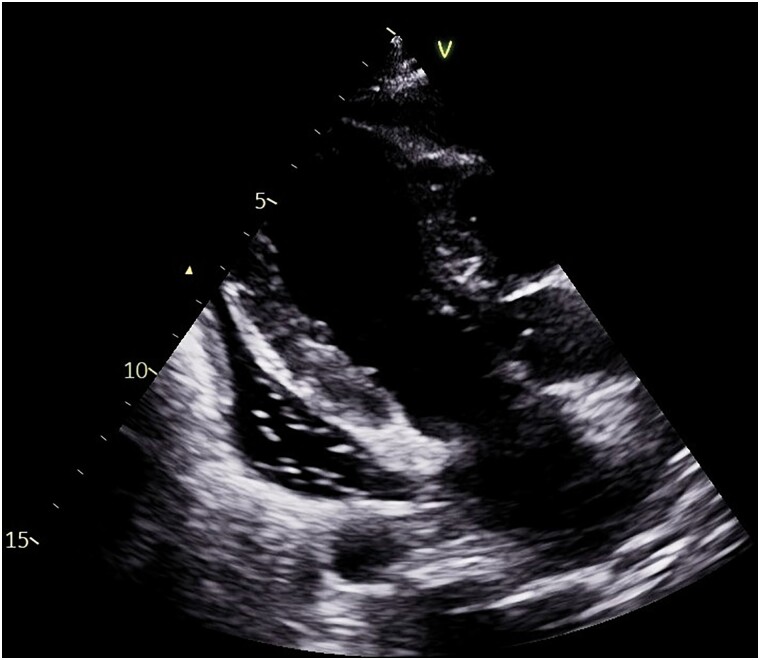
Parasternal long-axis findings in pneumohydropericardium. Parasternal long-axis view showing the moderate pericardial effusion (21 mm) with clear visualization of air bubbles posterior to the left ventricle.
